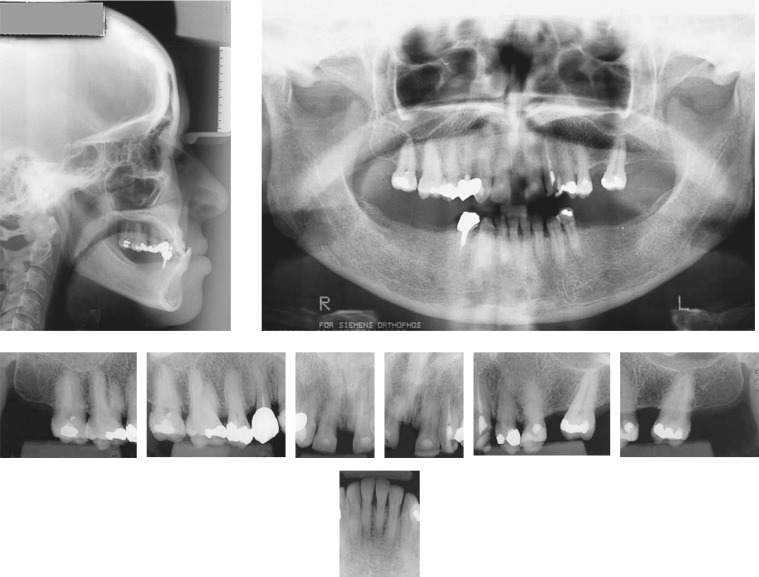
Initial lateral cephalometric radiograph, panoramic and periapical
